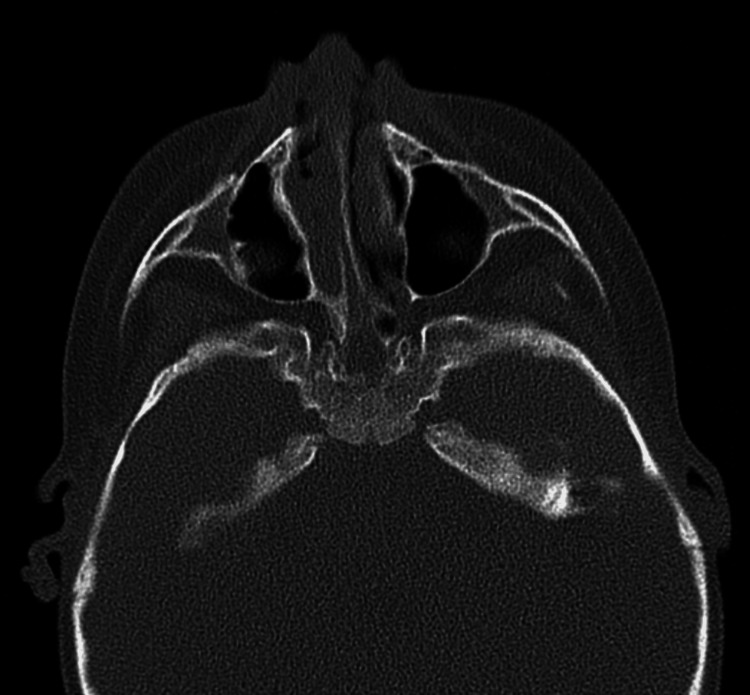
Bilateral choanal atresia This is an axial view CT scan of a one-month-old male infant showing right bony choanal atresia and left mixed choanal atresia
